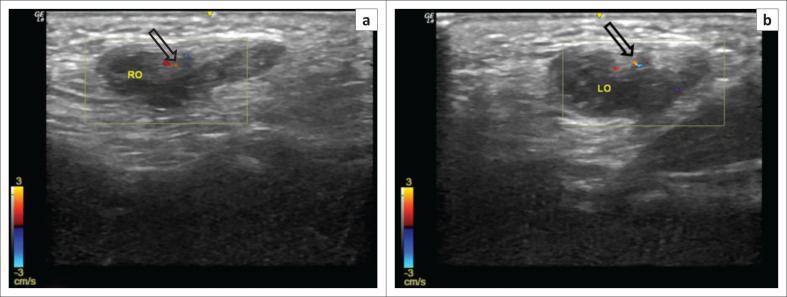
Colour Doppler ultrasound images (a and b) of the herniated contents show preserved vascularity (void black arrow) in the right (a) and left (b) ovaries.
